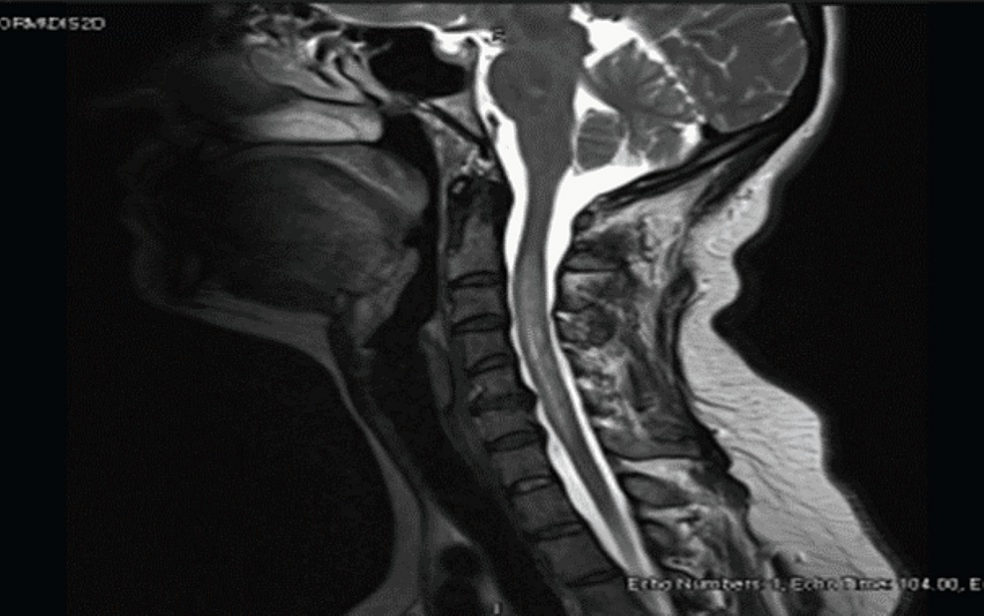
MRI of the cervical spine A long segment of heterogeneously bright T2 signal intensity in the cervical spinal cord extending from C2 down to C7 vertebral level with mild cord expansion. MRI: Magnetic resonance imaging.
